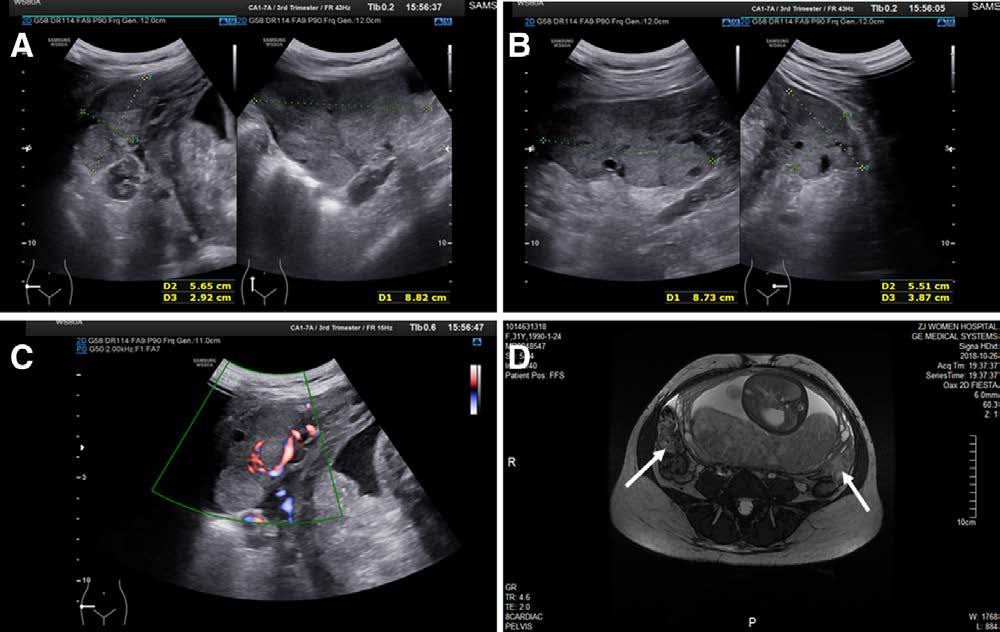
Sonography and magnetic resonance imaging of the ovarian masses. (A) Sonography of left maternal adnexal mass about 8.7 × 5.5 × 3.9 cm. (B) Sonography of right maternal adnexal mass about 8.8 × 5.7 × 2.9 cm. (C) Color Doppler evaluation demonstrated prominent arterial and venous flows. (D) The MR images show bilateral adnexal mass, mainly solid, but many vesicles were visible. MR = magnetic resonance.
